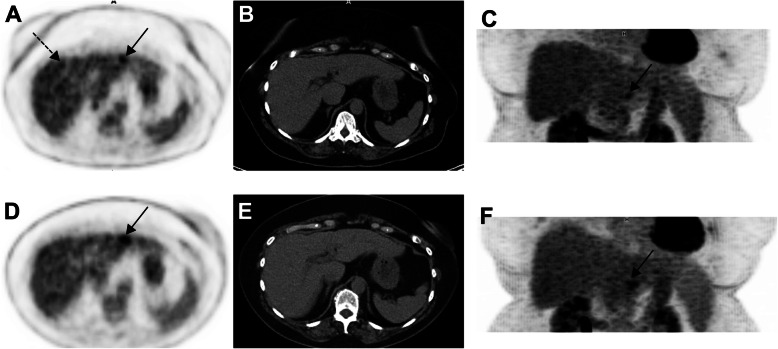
63-year-old woman with choroidal melanoma. The patient’s body mass index was 32.5. ^18^F-FDG PET images (axial slice A, zoomed MIP view B) show an indeterminate ^18^F-FDG focus located in the left liver lobe, segment 2 (black arrows) slightly more intense than the surrounding background seen in the right liver lobe (A, dotted black arrow). Given that liver metastasis is the main pattern of spread of uveal melanoma, follow-up was performed at 3 months and confirmed metastatic liver disease (D, E and F, black arrow)
